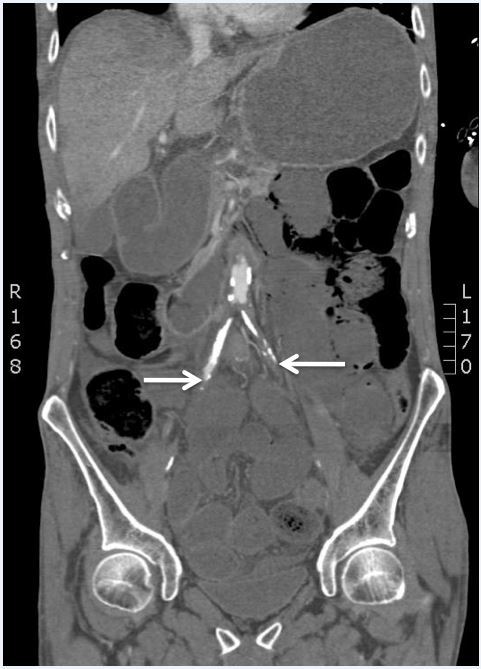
Coronal computed tomography demonstrating aortoiliac vascular occlusion.
